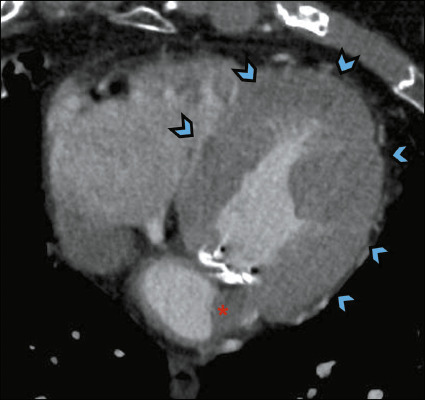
CTA for TAVR planning in a 73-year-old male patient, showing concentric hypertrophy
